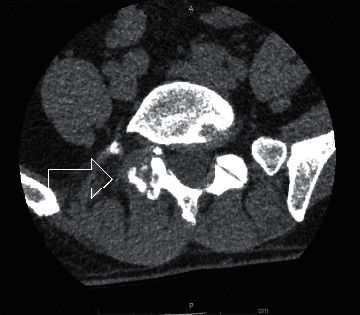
CT of the lumbar spine showing erosive degenerative changes of the right L5-S1 facet joint (arrow), with mild soft tissue prominence surrounding this region. Narrowing of the right L5-S1 neural foramina is also appreciated.
